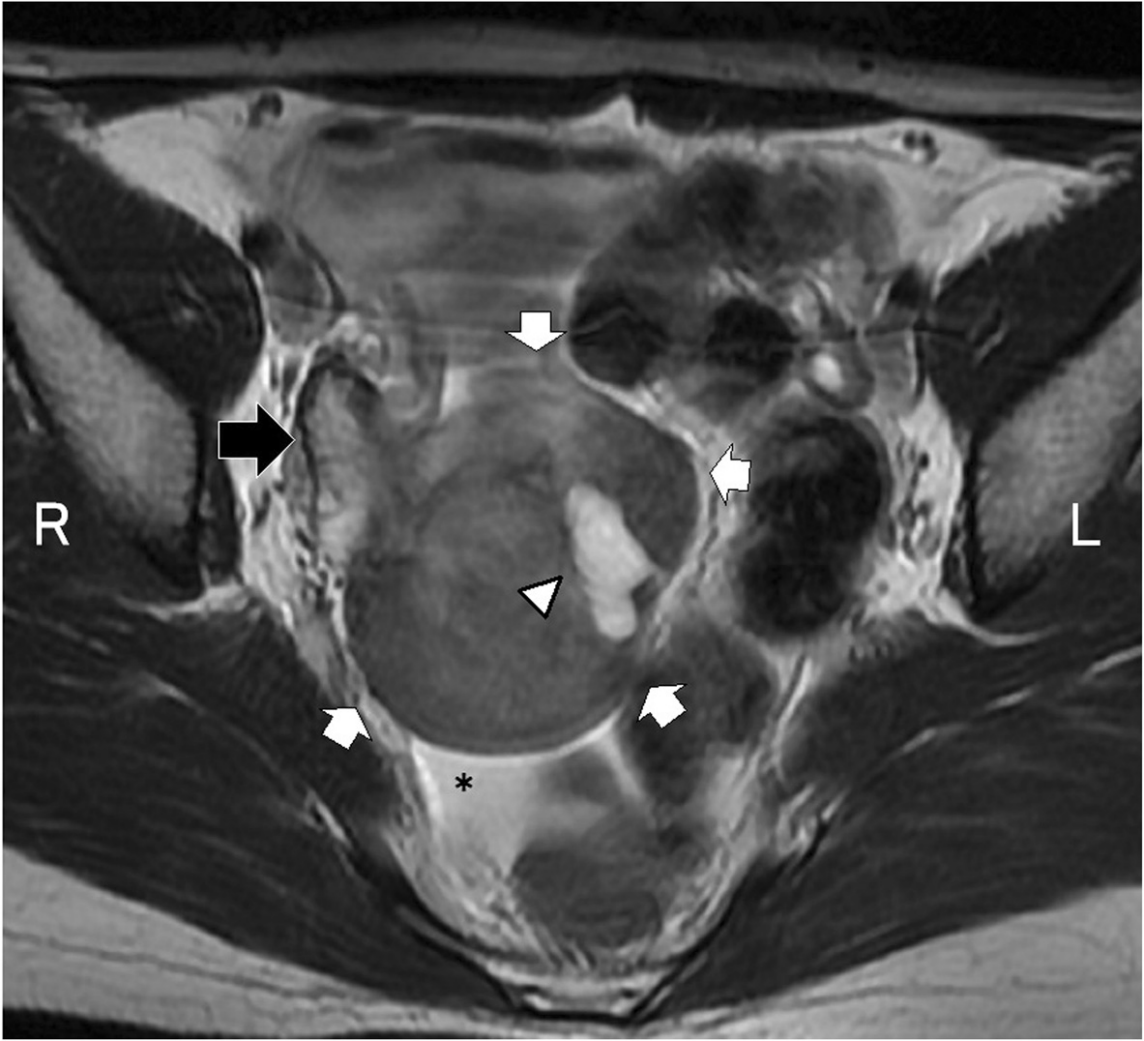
MRI appearance of the tumor. Axial T2-weighted MR image showed a well-circumscribed mass (white arrows) including cystic component (arrowhead) adjacent to the right ovary (black arrow) as having inhomogeneous low signal intensity. Small amount of ascites was noted (*)
